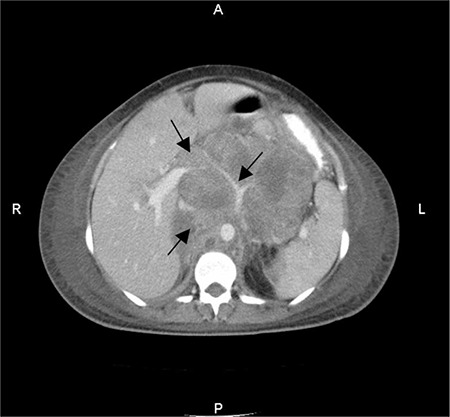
Axial contrast-enhanced computed tomographic image reveals a 13x11 cm mass located between the liver and para-aortic area
